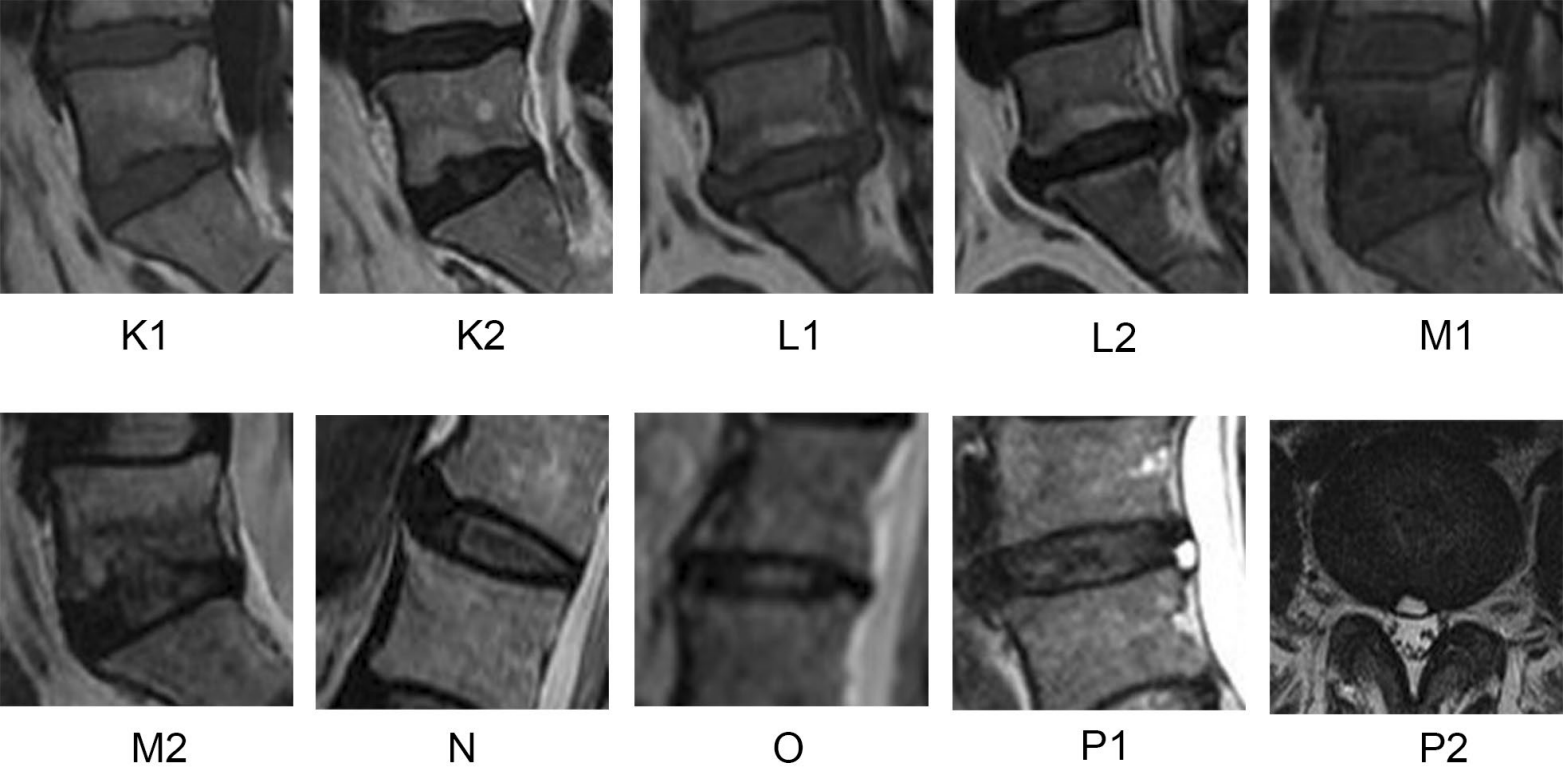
MRI of the Lumbardiscs. K1 (Modic change type I on T1-weighted image), K2 (Modic change type I on T2-weighted image), L1 (Modic change type II on T1-weighted image), L2 (Modic change type II on T2-weighted image), M1 (Modic change type III on T1-weighted image),M2 (Modic change type III on T2-weighted image), N (Spondylosis deformans), O (Pure decreased disc height), P1 (Mid-sagittal viewof High intensity zone, HIZ), P2 (Axial viewof High intensity zone, HIZ)
